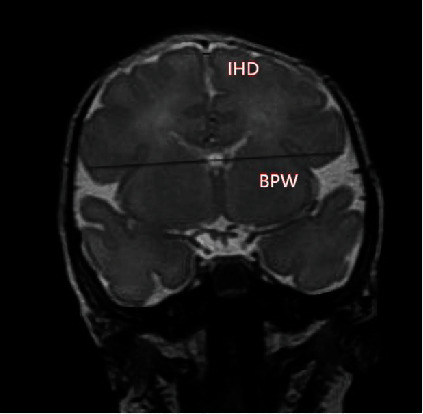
cMRI. Routine cranial MRI (cMRI) was performed at 37 weeks of PMA to measure the BPW and IHD. All preterm infants were given intravenous injection of phenobarbital sodium (5 mg/kg) 30 minutes before the cMRI examination and wrapped with a cotton quilt. The examination was performed when the infants were asleep. All cMRI data were acquired and recorded by two radiologists without knowledge of the clinical situation. IHD: interhemispheric distance; BPW: biparietal width.
